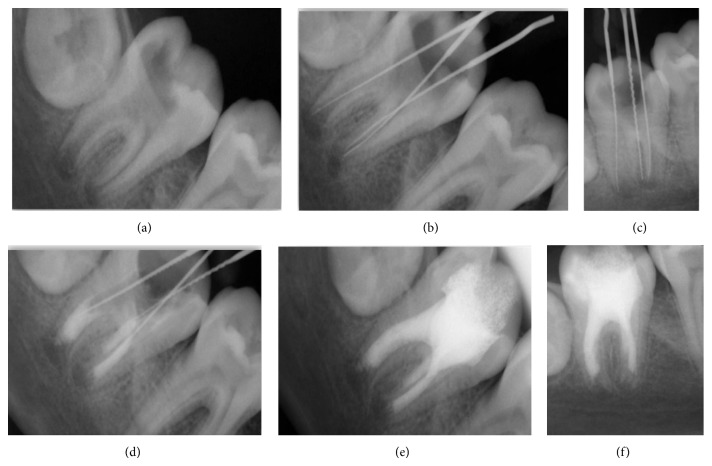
(a) Preoperative. (b) and (c) Working length determination. (d) MTA apical plug of 4-5 mm thickness. (e) Final obturation. (f) After 8-month reassessment, continuous lamina dura and consistent width of periodontal ligament space suggest healing of the periapical lesion.
